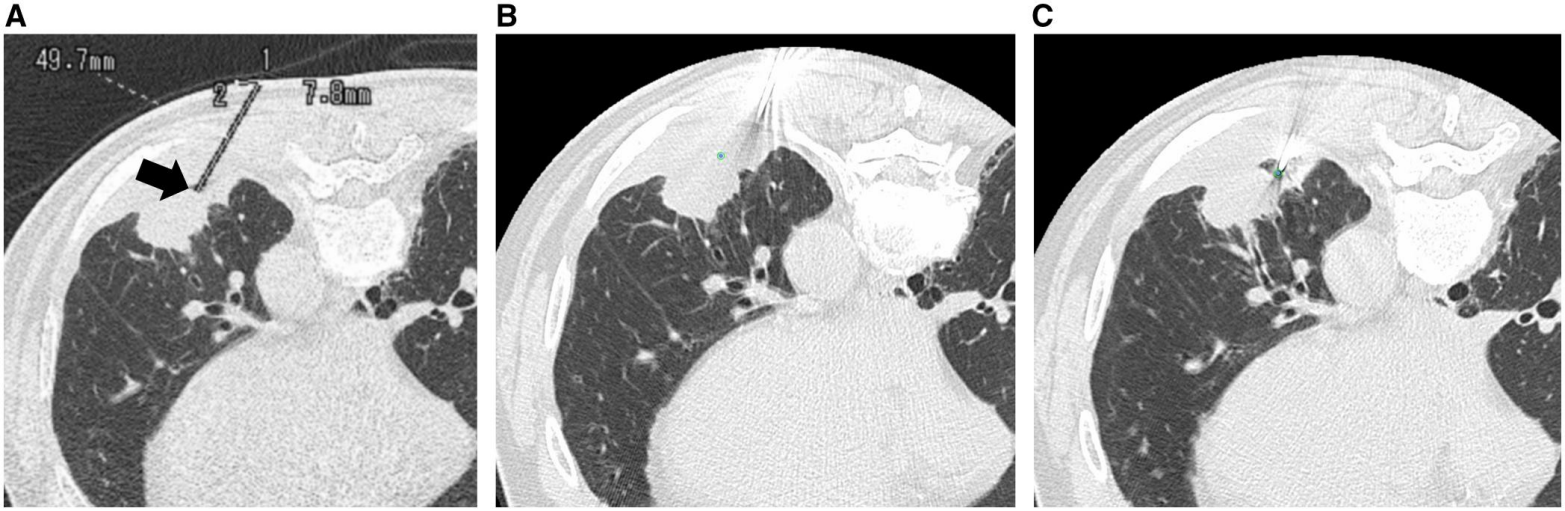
(A) Screen capture of the axial CT image (1 mm thickness) before needle insertion showing the planned needle tract line. The distal end of the needle tract line (arrow) represents the target point. (B) Axial CT image (1 mm thickness) after needle insertion. The target point (circle) is defined based on the screen capture of the axial CT image before insertion (A). The CT coordinates of the target point are *X*: 25.91 mm, *Y*: −8.96 mm, *Z*: −1688.4 mm. (C) Axial CT image (1 mm thickness) after needle insertion. The needle tip (circle) is defined. CT coordinates of the needle tip are *X*: 18.58 mm, *Y*: −11.7 mm, *Z*: −1684.4 mm. The accuracy of the needle insertion (ie, 3-dimensional Euclidean distance between the target point and the needle tip) was calculated to be 8.8 mm.
